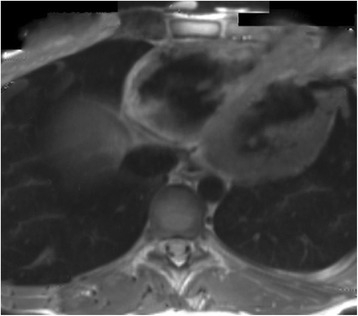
A case of a 14 year old boy from Spain, who was admitted into emergency room after being resuscitated from cardiac arrest, secondary to malignant ventricular tachycardia that developed while he was playing basketball. Four months later he was brought up again to the emergency room after collapsing during mild exercise. His MRI revealed more structural and functional anomalies of the right ventricle, with more myocardial loss and more involvement at layers of posterior and apical walls. The left ventricle was slightly affected. Original materials from an open access article [58]
